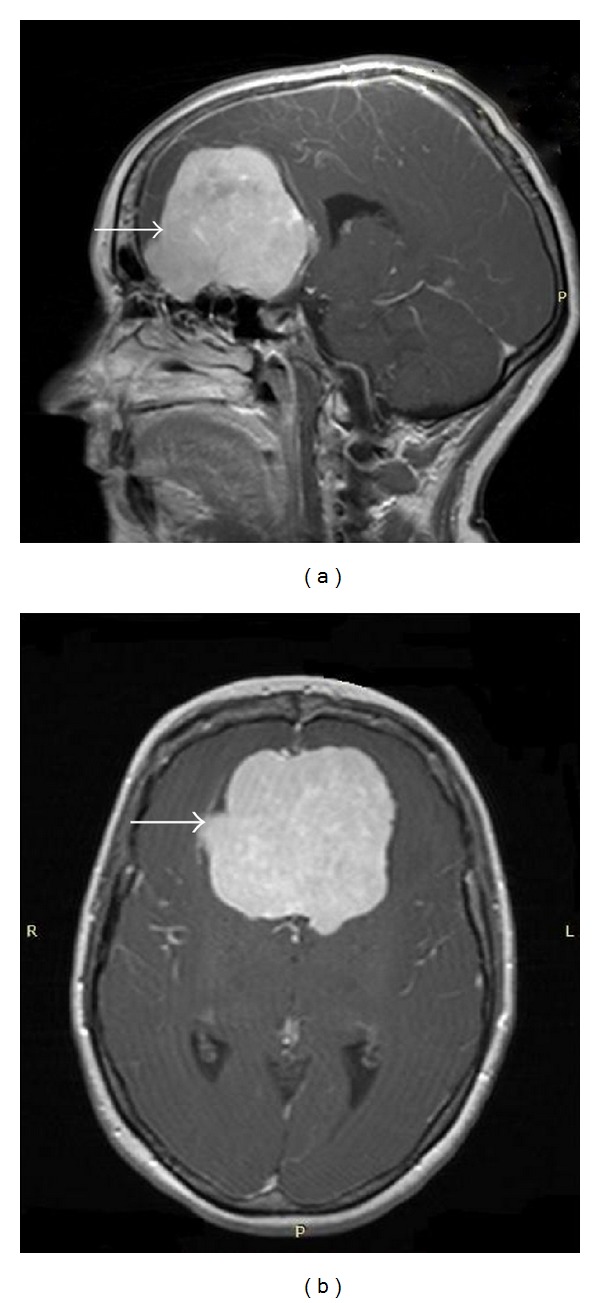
Arrows show a large lobulated extra-axial tumor compressing the olfactory sulcus in gadolinium-enhanced T1-weighted MRI.
